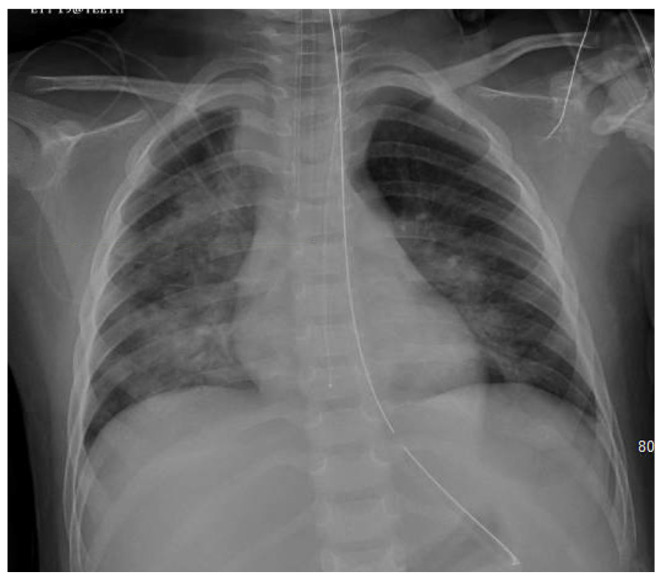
AP portable chest X-ray of a 6-year-old boy with acute respiratory failure and fluid overload showing bilateral perihilar patchy opacities.
